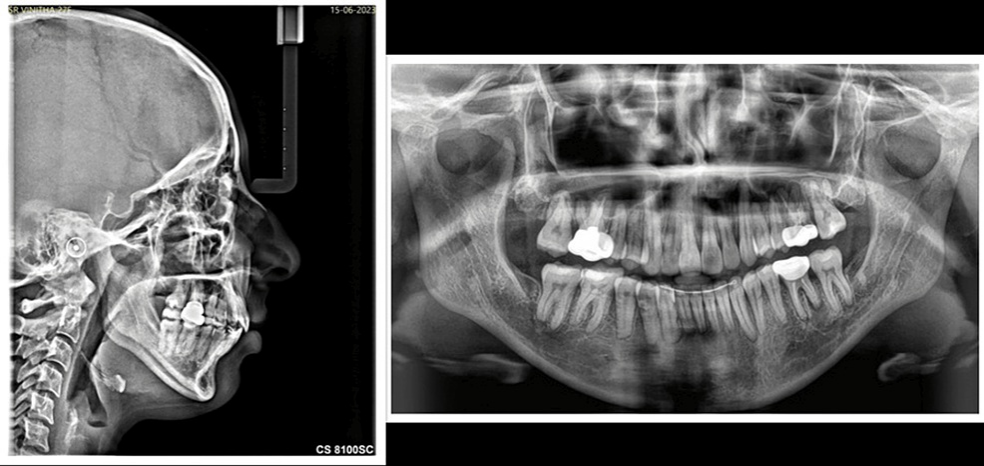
Posttreatment radiographs U1-SN: 112.777 degree; L1-Apog: 6 mm; L1-NB: 30.777 degree; L1-NB: 9 mm
